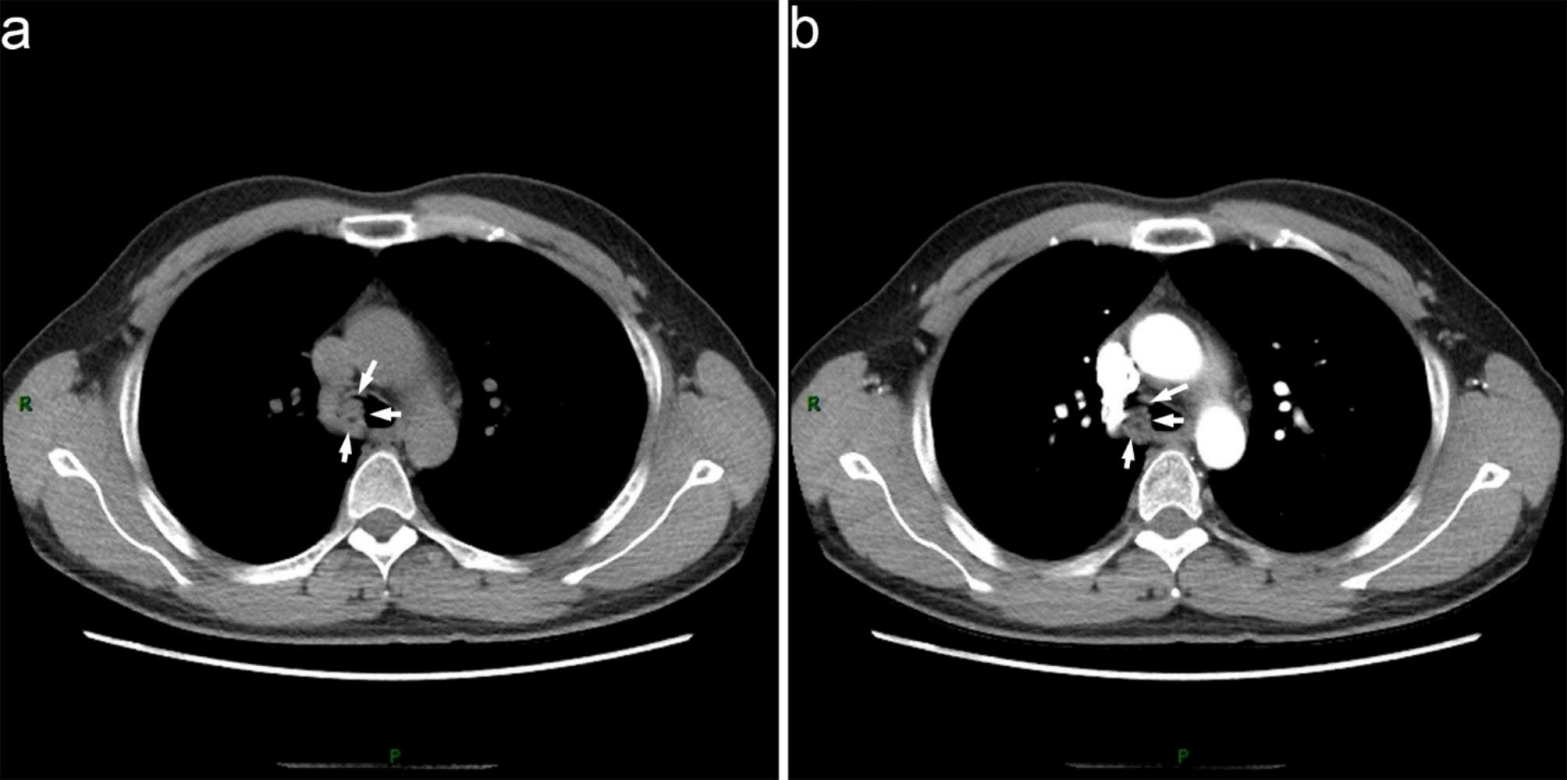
CT of chest showed the nodule. (a) The nodule (white arrows) on the membranous portion of the right main bronchus. (b) The nodule (white arrows) without enhancement on the enhanced chest CT.
